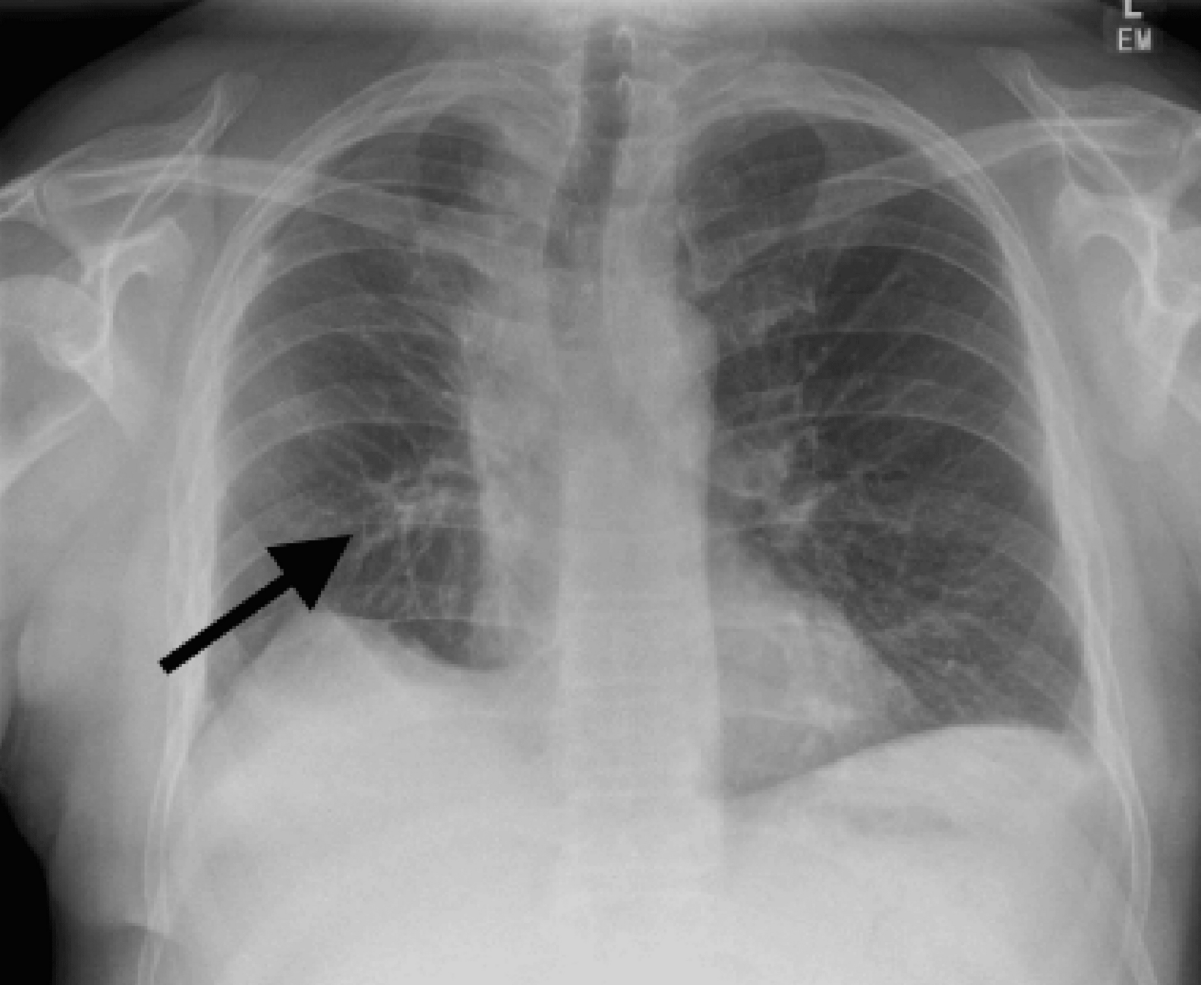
Chest X-ray in PA view, erect, post-operative, demonstrating volume loss in the right hemithorax (black arrow). PA: posteroanterior view.
